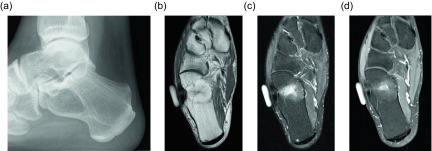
Intraosseous lipoma: 48-year-old male with lucent lesion in the calcaneus on prior radiographs, status post aspiration. Suspected lipoma versus bone cyst. Lateral radiograph (a) of the ankle demonstrates radiolucent lesion at the anterior aspect of the calcaneus. Axial PD (b), PD FS (c) and axial T1 FS post-gadolinium (d) images. The lesion is predominately isointense to fat with loss of signal on fat saturated images. Foci of high signal within the lesion on the PD FS images represents cystic change. On post contrast imaging, mild enhancement of the cystic portions is observed.
